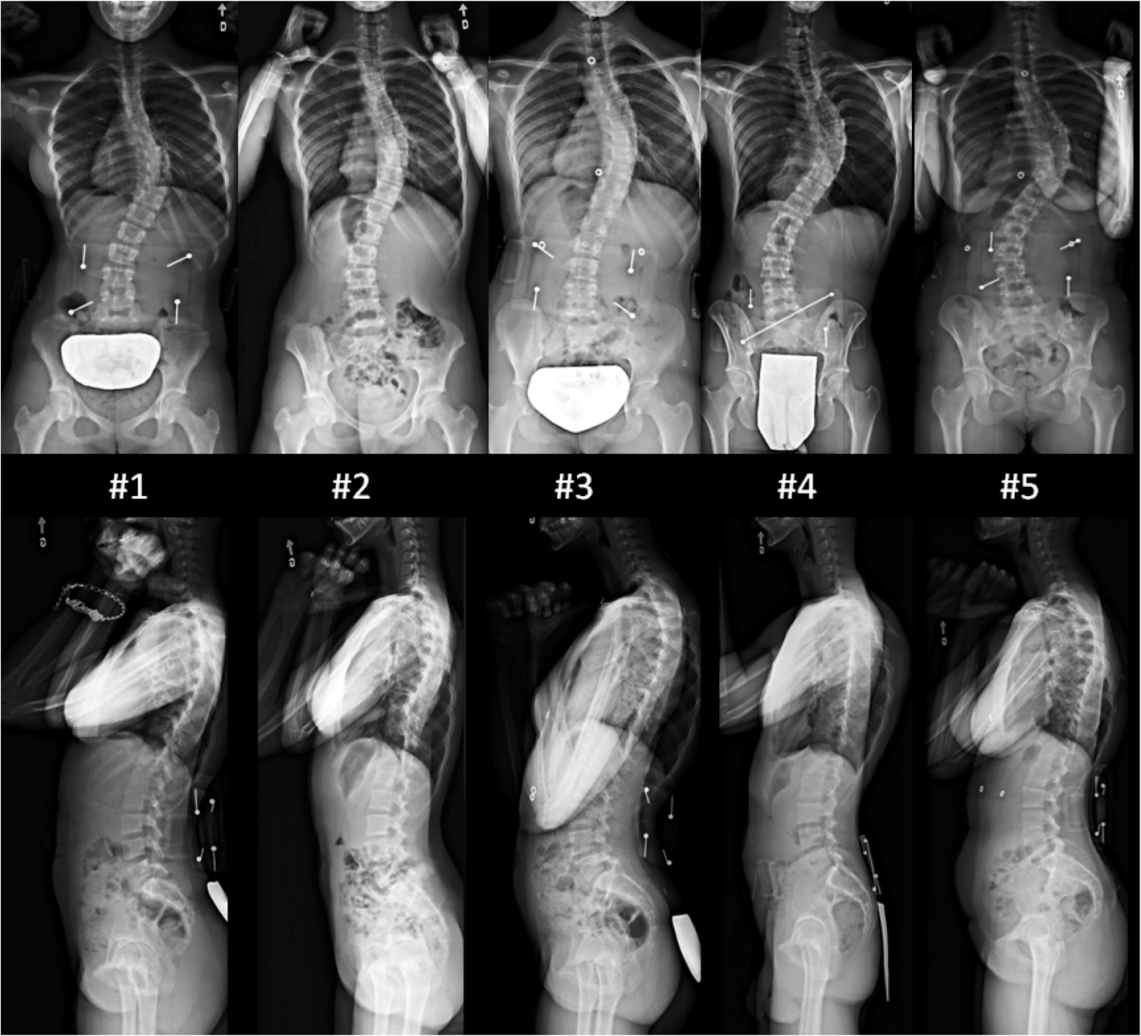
Preoperative standing posteroanterior and lateral radiographs of the five cases
